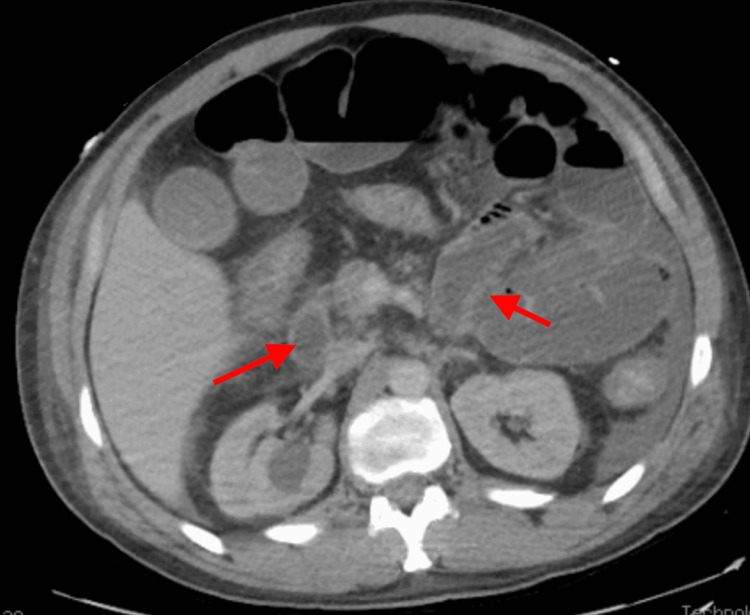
Axial CT abdomen showed diffuse bowel wall thickening with abnormal enhancement (arrows).
